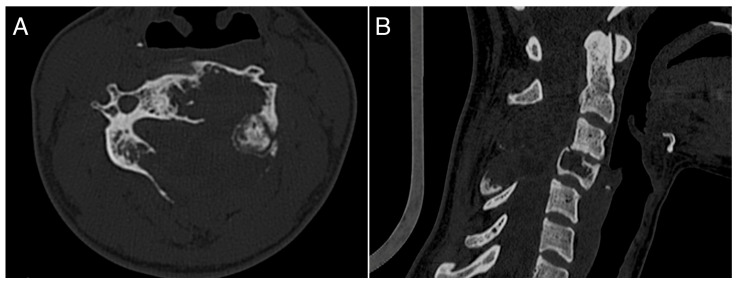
CT scan showing a local recurrence occurring ten months after stopping denosumab treatment. (A): axial view; (B): sagittal view.
